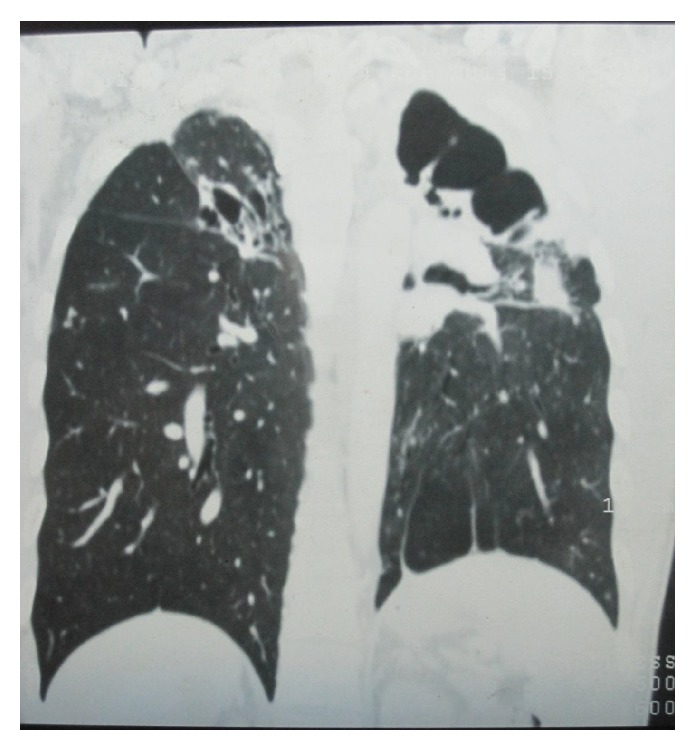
CT scan thorax showing multiple cavitary lesions in left lung predominantly in upper lobe, with hemorrhagic debris in the cavities. Bronchiectatic changes were also noted in right lung but to a milder level of affection.
